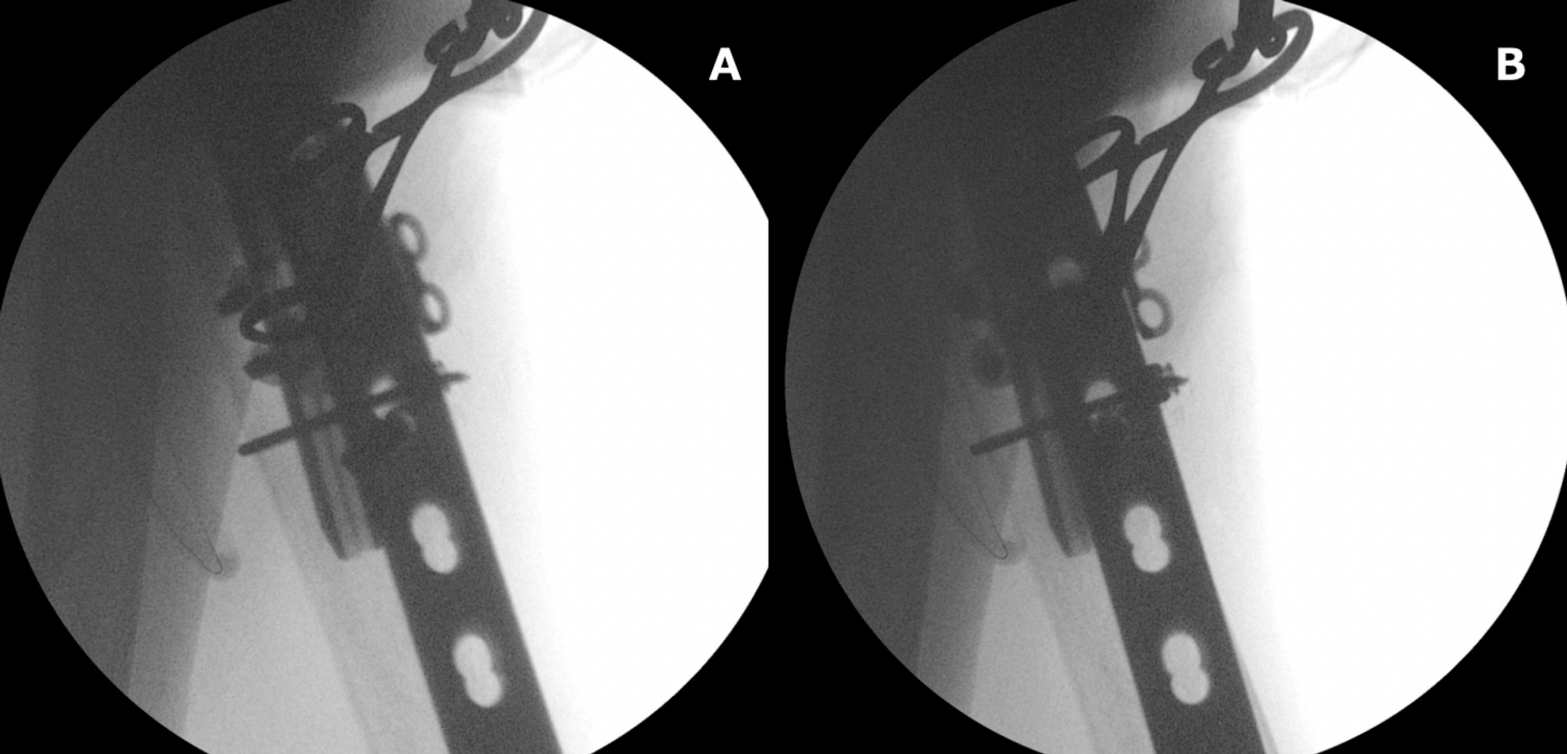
Intraoperative radiographs depicting the screw has been inserted into the bicortex A: lateral view; B: lateral view with a slightly different angle
